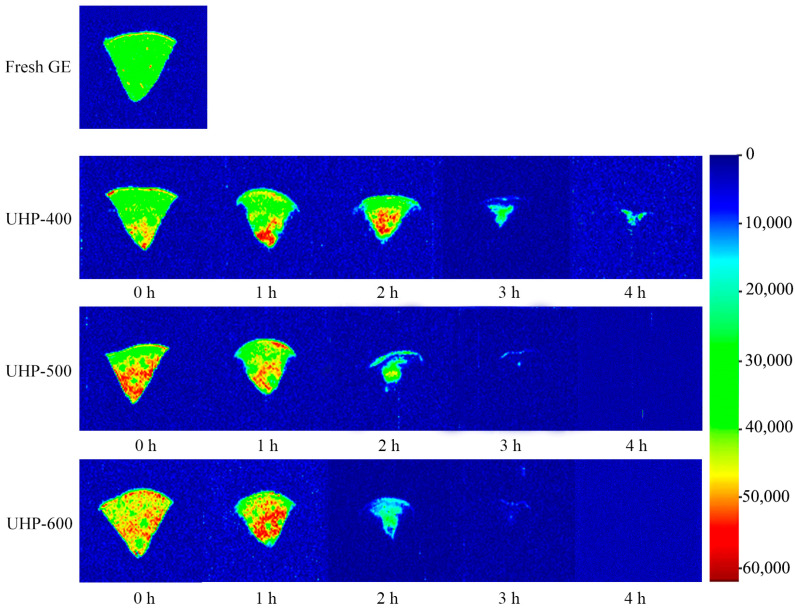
MRI images of GE samples under different UHP pre-treatment dried at 60 °C.
